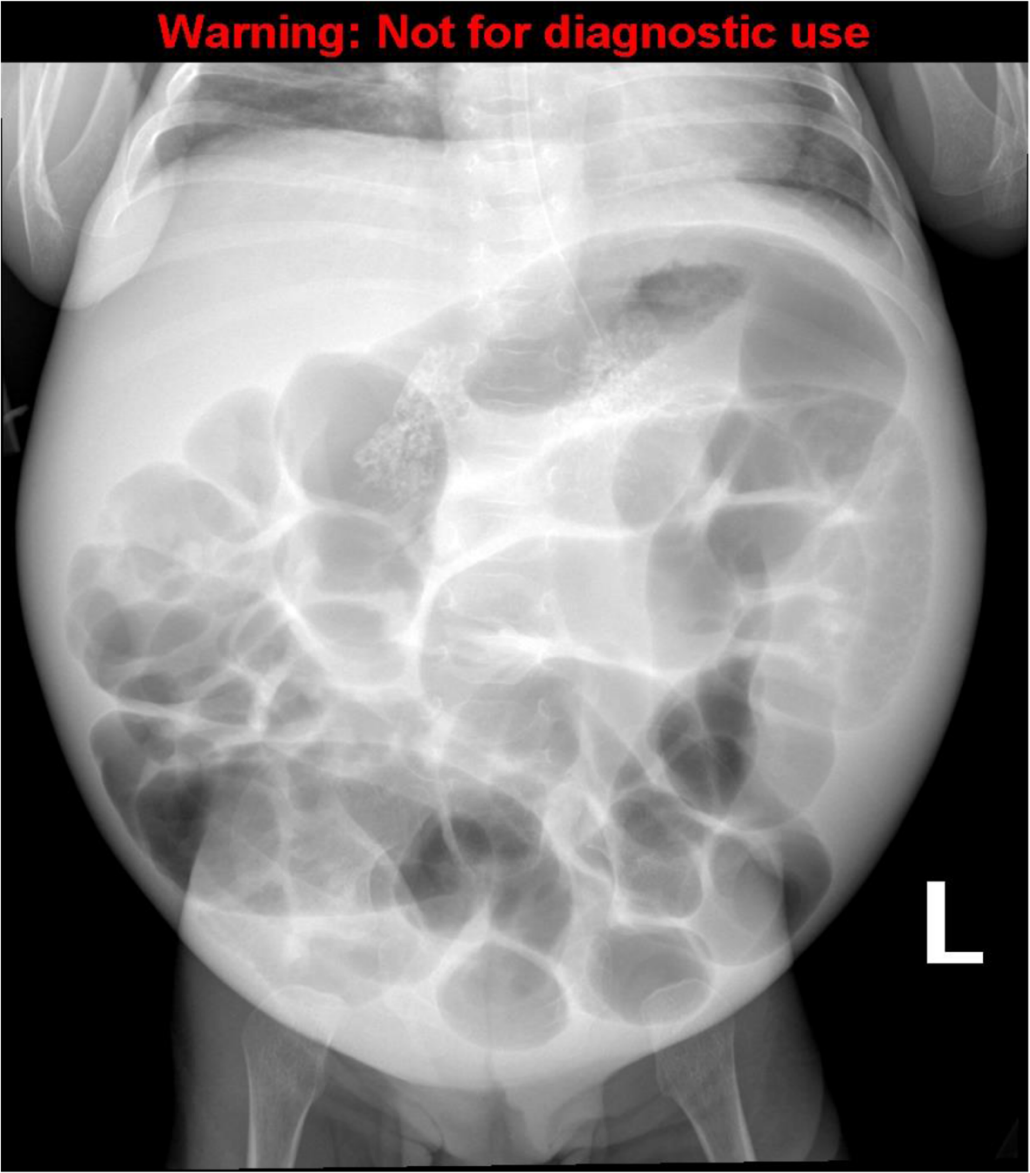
Plain abdominal X-ray showing bilateral adrenal calcification
